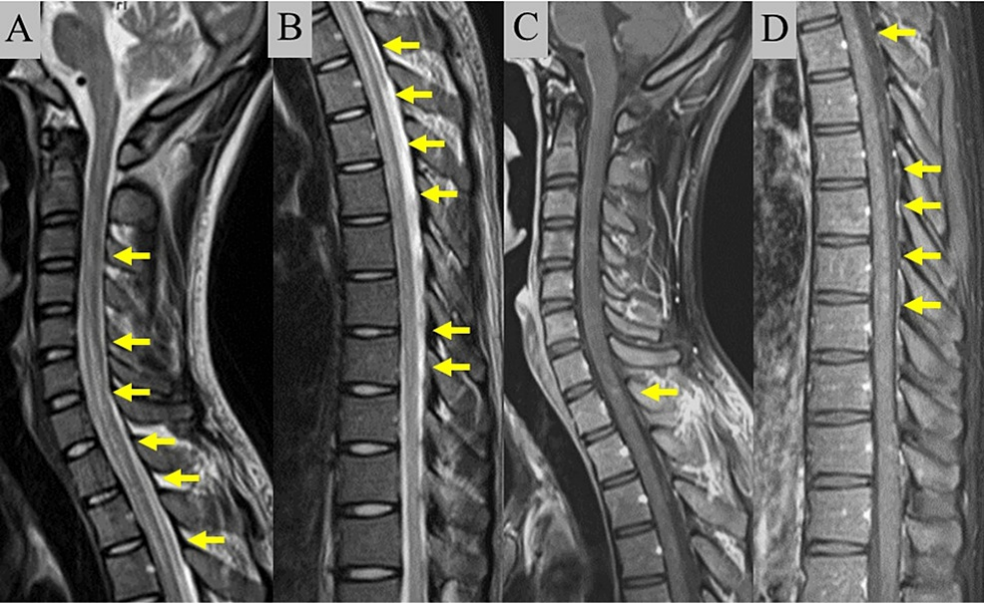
Diagnostic findings of myelin oligodendrocyte glycoprotein antibody-associated disease (MOG-AD). A and B: T2-weighted imaging of the spinal cord revealed a long lesion in the C2-T8 region (arrow). C and D: Gadolinium-enhanced T1-weighted imaging revealed a partially enhanced lesion in the C2-T8 region (arrow).
